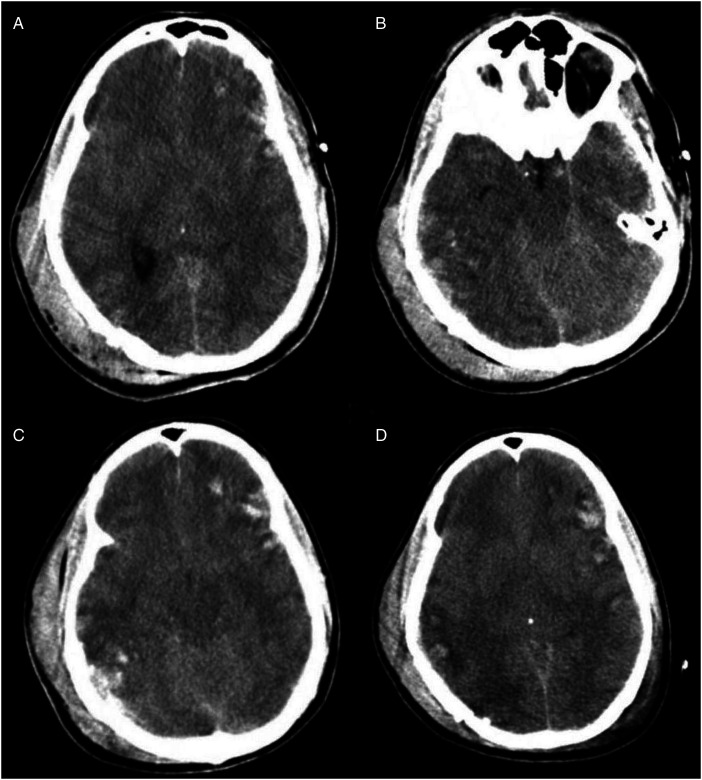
Four views from computerized tomography (CT) scans of the head from 1.8 h after injury to 44.7 h after injury. At 1.8 h, there are hemorrhagic contusions in the right temporal and posterior parietal regions, as well as in the left frontal and temporoparietal regions (A, B). Similar findings are present on follow-up CT scans at 12.9 h (C) and 44.7 h (D).
